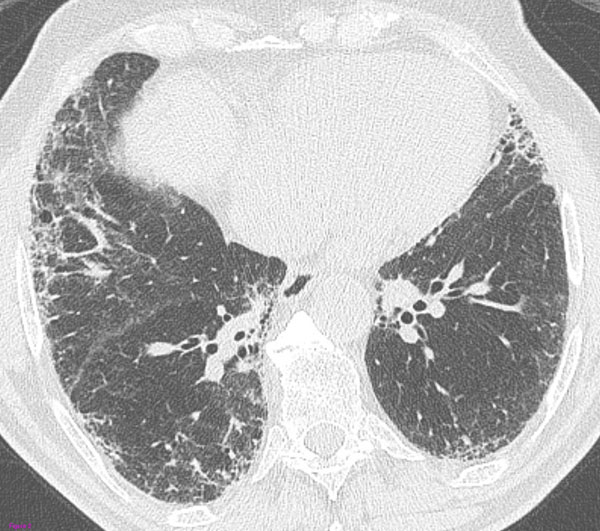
Biopsy-proved UIP with a possible UIP pattern at HRCT. The transverse HRCT image through the lower lobes shows patchy and peripheral reticular opacities without obvious honeycombing.
